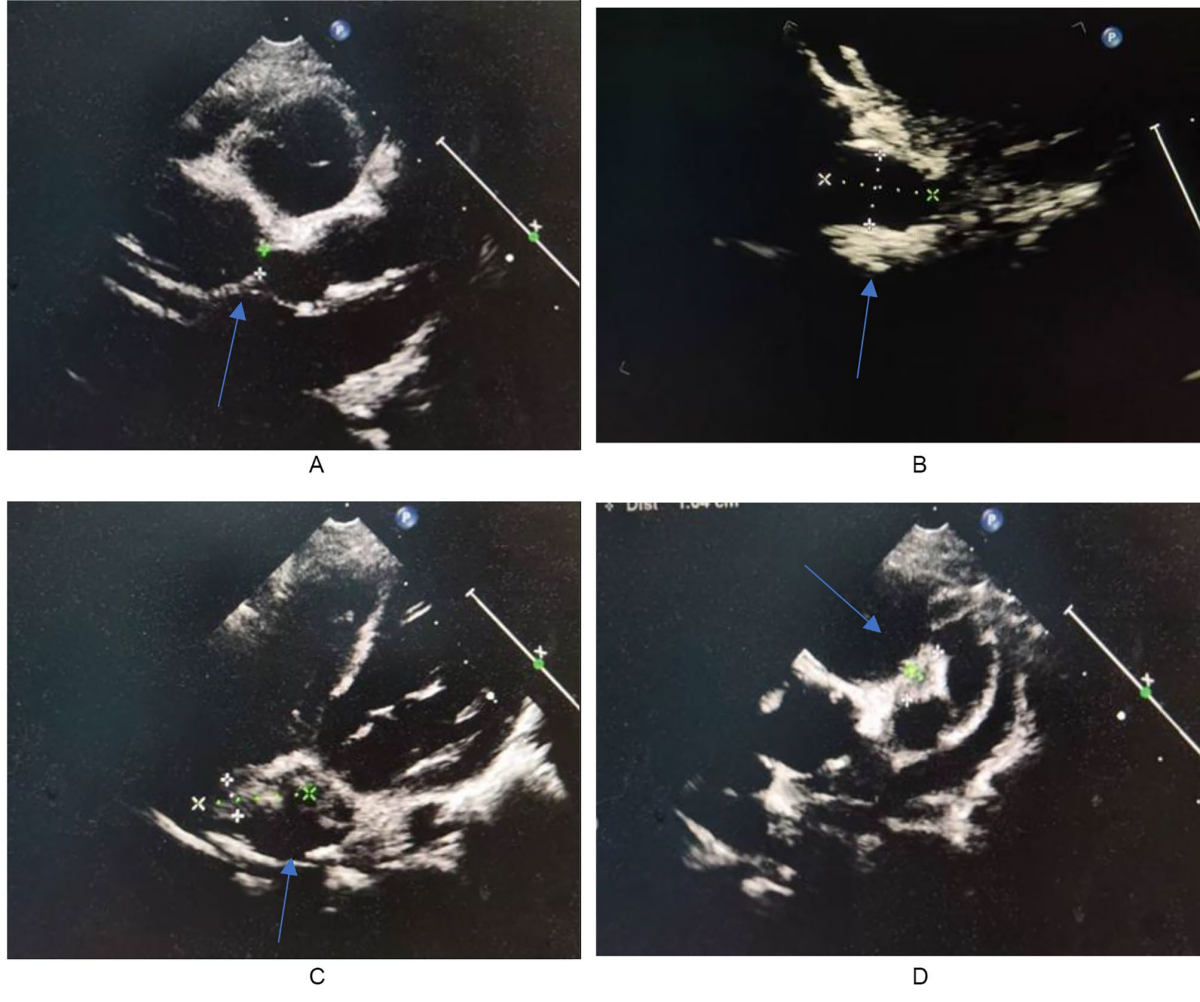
A Ultrasound showed aneurysmal dilation of the left main coronary artery in Patient(P) 8 (arrow). B Ultrasound showed aneurysmal dilation of the left main coronary artery in P9 (arrow). C Ultrasound showed right coronary artery thrombosis in P8 (arrow) D Ultrasound showed thrombosis in the left anterior descending coronary artery in P8 (arrow)
